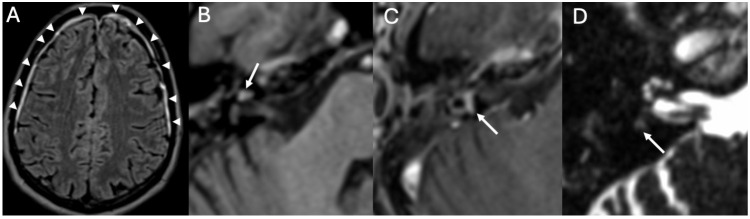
A case of inner ear hemorrhage in a 32-year-old patient with Pneumococcal Meningitis. Axial enhanced FLAIR (A), unenhanced T1 (B), enhanced T1 (C), and heavily T2-weighted sequences (D) show diffuse pachymeningeal enhancement (arrowheads in (A)), spontaneous T1 hyperintensity of the right cochlea (arrow in (B)) caused by hemorrhage and contrast-enhancement of ipsilateral lateral semicircular canal (arrow in (C)), which has become fibrotic after two months (arrow in (D)). Image readapted from [36].
